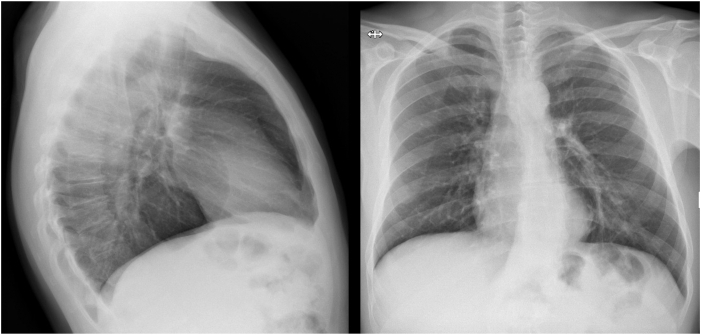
Left-lateral (top) and posteroanterior (bottom) chest radiographs show a bullous lesion in left-posterior mediastinal region.
